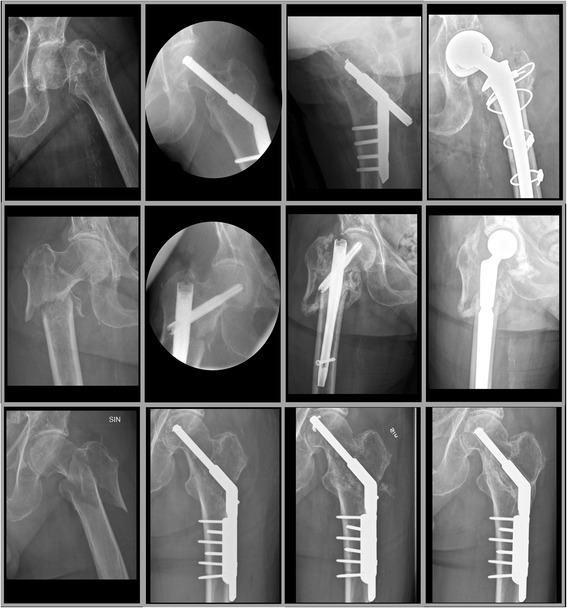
From left to right preoperative, intra/postoperative, post technical complication and final radiographs of the typical and most frequent type of technical complication that occurred with dynamic hip screw (medialisation and nonunion followed by hip joint replacement, upper panels), intramedullary nail (nonunion followed by hip joint replacement, middle panels) and uniaxial Medoff sliding plate (Twin hook penetration followed by Twin hook replacement and fracture healing, lower panels)
